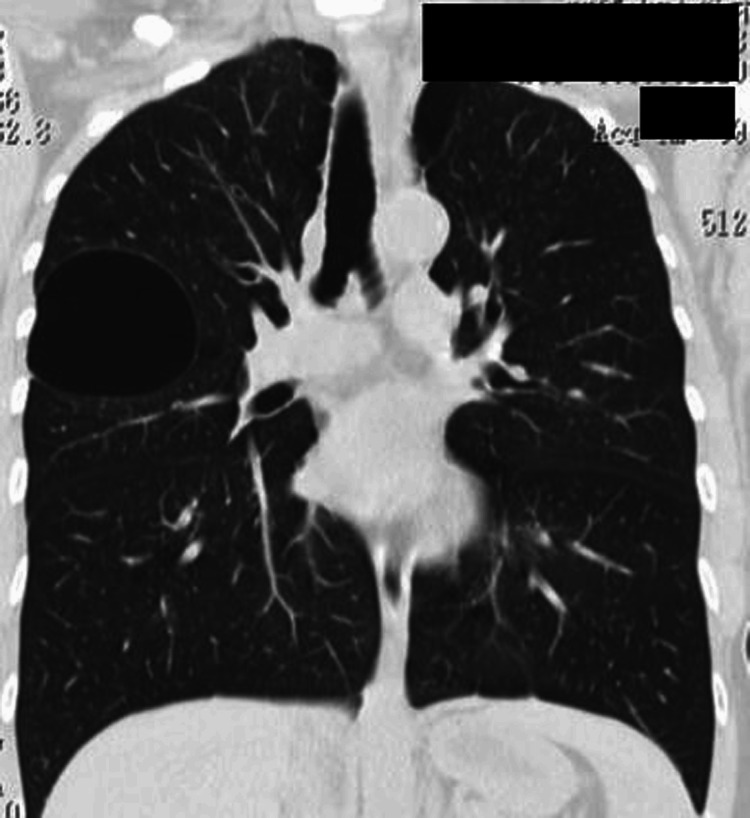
Coronal CT on initial presentation An air-filled cyst (9 cm) is seen in the apicoposterior segment of the right upper lobe with no fluid or consolidation.
